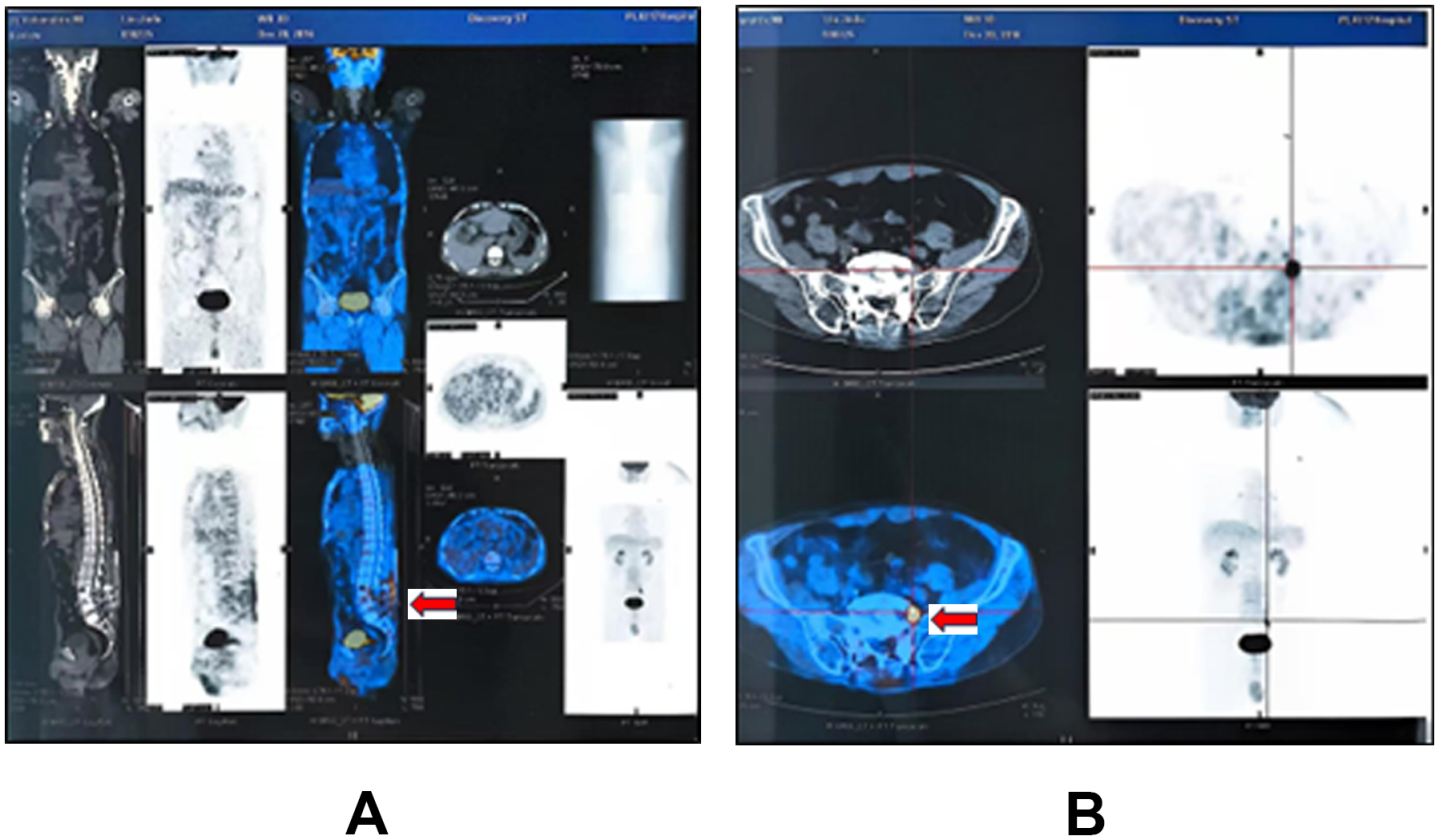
Postoperative PET-CT (December 2016): (A) Sagittal view showing patchy areas of moderately increased radiotracer uptake, with a maximum standardized uptake value (SUVmax) of 4.46 within the soft tissues and bone at the surgical site. (B) Axial view at the L5 level revealing thickening of the left L5 nerve root, more pronounced at the intervertebral foramen exit, with a SUVmax of 12.92.
